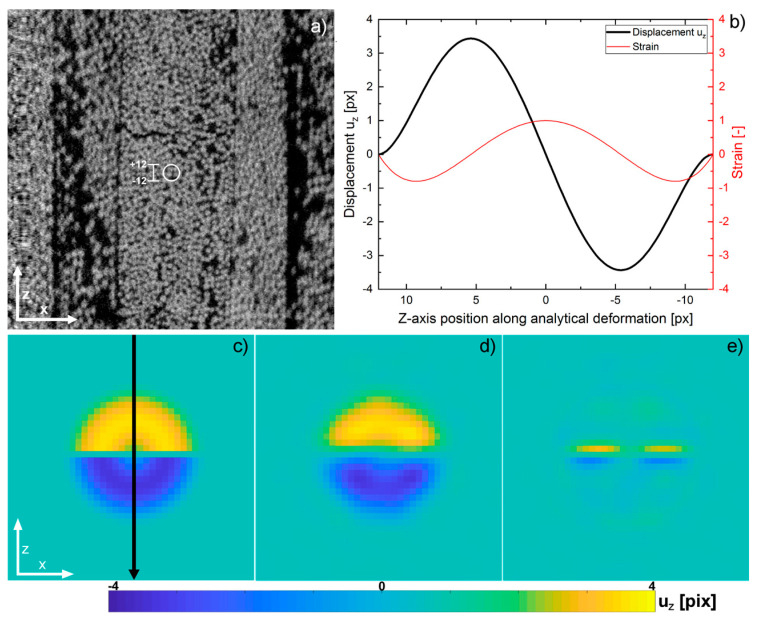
The region of the analytical deformation is marked in the reference CT slice (a). The displacement within this deformation ranges from −3.5 to +3.5 px with a max. strain of one in the center. The graph (b) shows a line plot through the center of the analytical deformation. The central xz-slice of the manufactured solution together with the position of the line profile (c), the displacement field obtained from DVC (d) and the residuals between the former (e).
